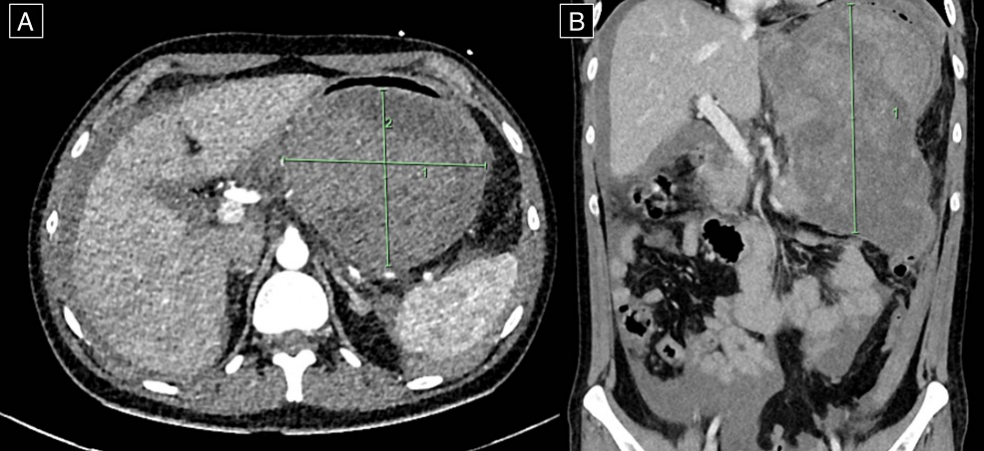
(A) Axial view of computer tomography (CT) of the abdomen showing a large encapsulated collection suggestive of walled-off necrosis measuring 105 × 120 × 178 mm posterior to the stomach. (B) Coronal view demonstrating the length of collection measuring 178 mm.
